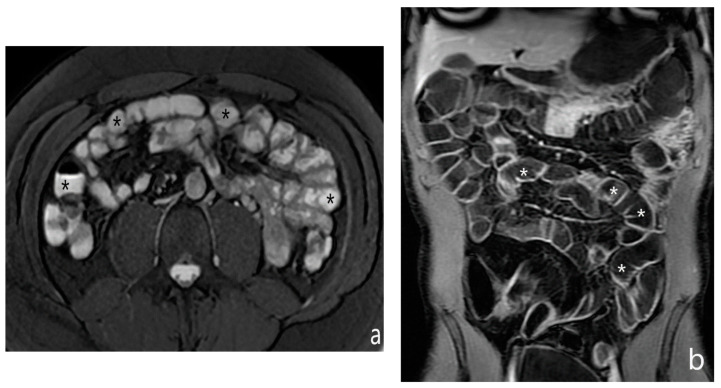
MR-E: example of intestinal loops’ distention by biphasic contrast medium (polyethylene glycol solution). Intestinal lumen (asterisks) is hyperintense in T2-weighted axial image (a) and hypointense in T1-weighted coronal image after gadolinium injection (b). Original figures by LMM and LL.
